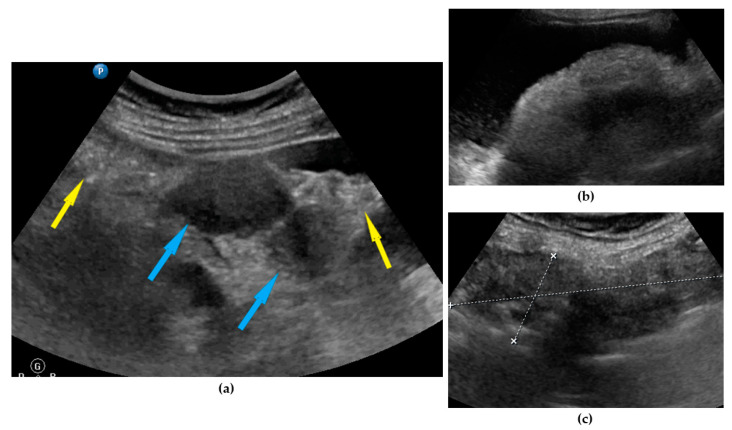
Ultrasound images of omentum involvement: (a) focal infiltration (blue arrows identify tumors, yellow arrows identify normal omentum); (b) diffuse omental infiltration with ascites; (c) diffuse omental infiltration without ascites.
